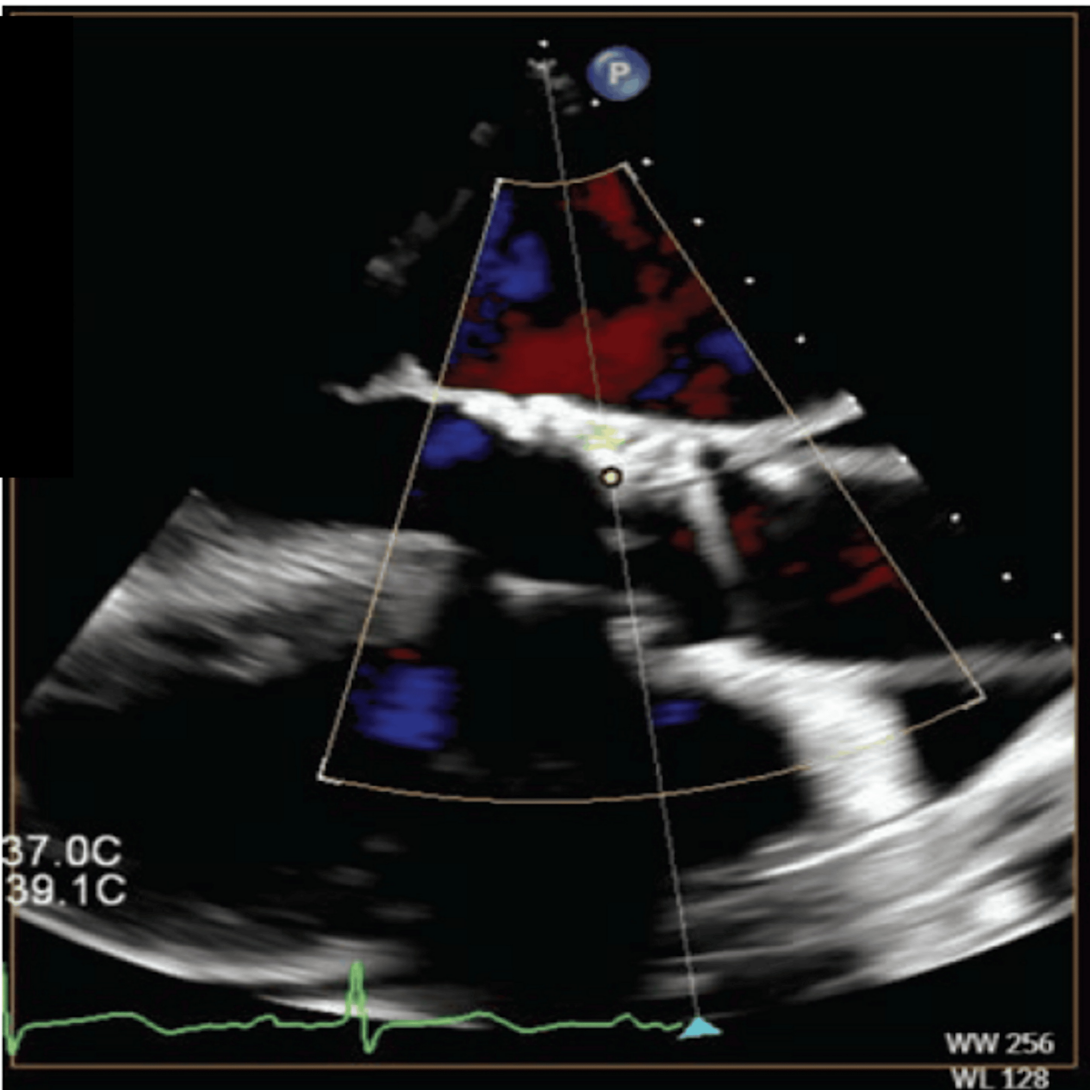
Transesophageal echocardiography (TEE) showing no paravalvular leak after successful deployment of the Amplatzer Vascular Plug. Figures reprinted from [12], ©2025 HMP Global. Used with permission.
